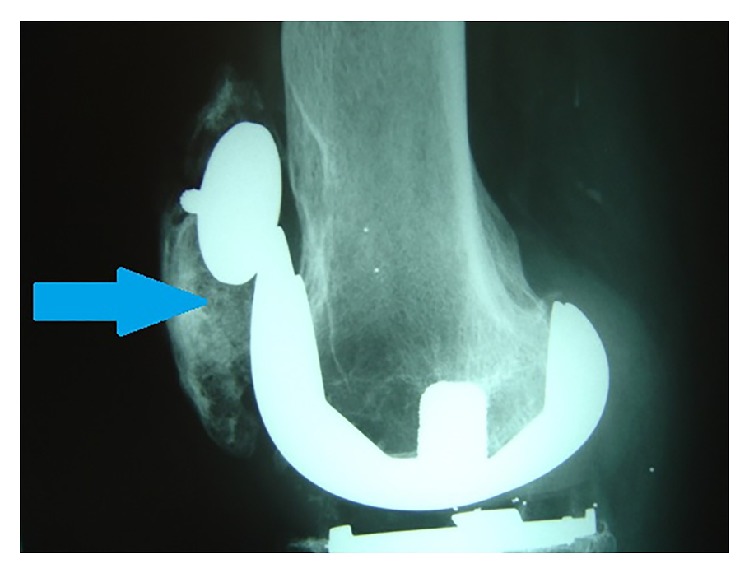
Lateral radiograph of the replaced knee demonstrates the loose patella prosthesis and surrounding osteolysis.
